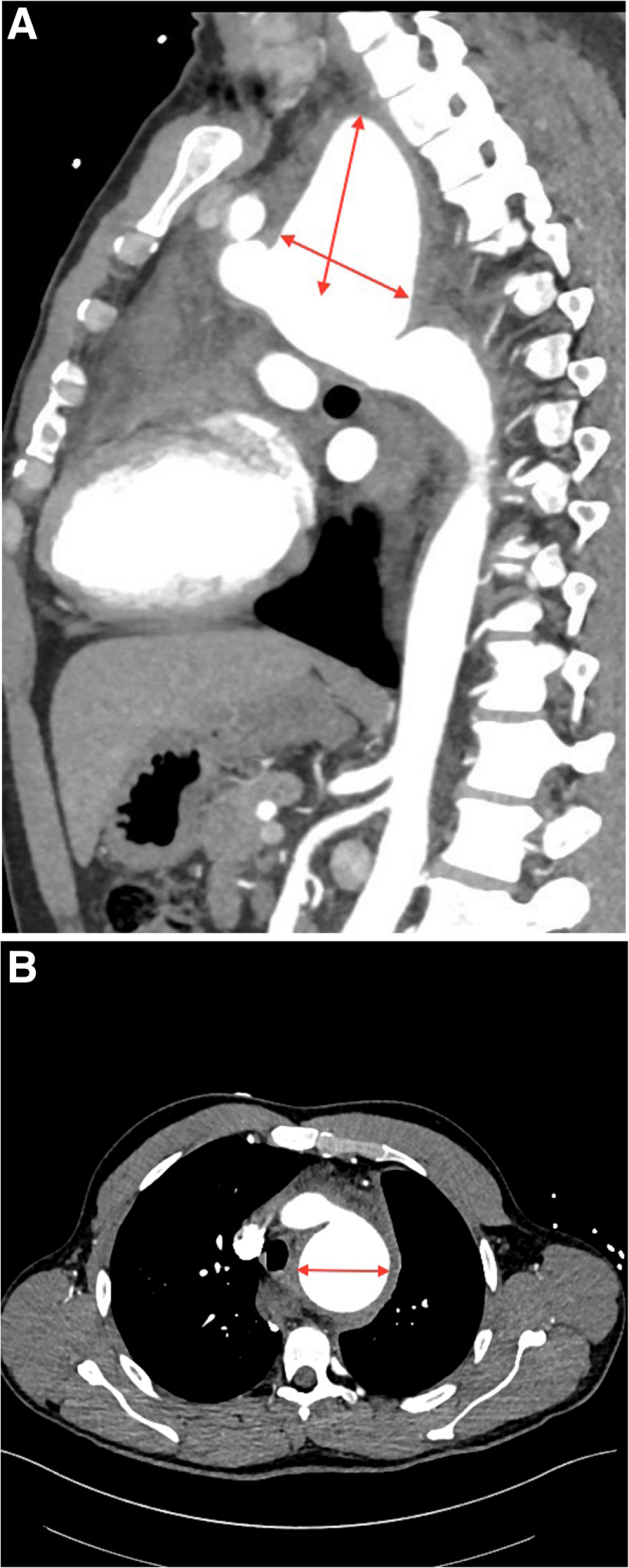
a Sagittal contract-enhanced CT-scan reconstruction demonstrated an 8.5-cm saccular aneurysm in the distal aortic arch. b Axial contract-enhanced CT-scan showing transversal diameter of Kommerell diverticulum with surrounding mediastinal hematoma suggesting diverticulum rupture
